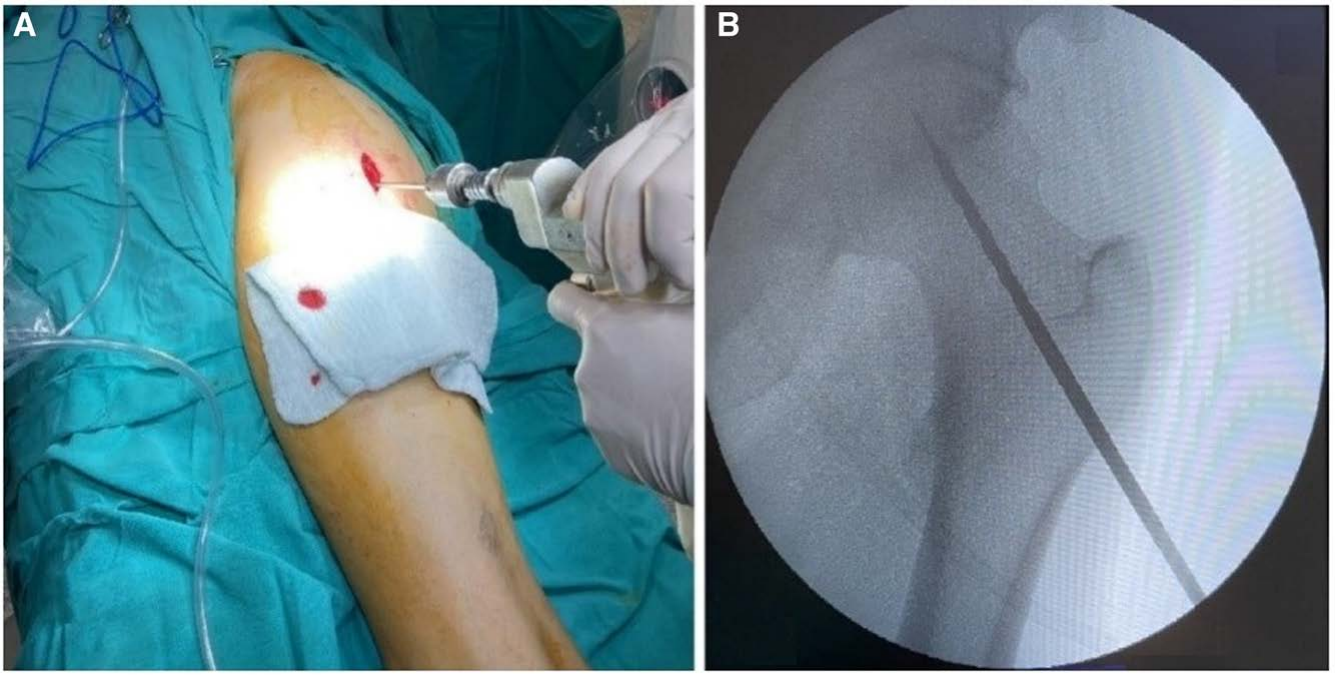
(A) Intraoperative surgical view of the incision site. (B) Anteroposterior X-ray view of the drilling via the guide K-wire.
